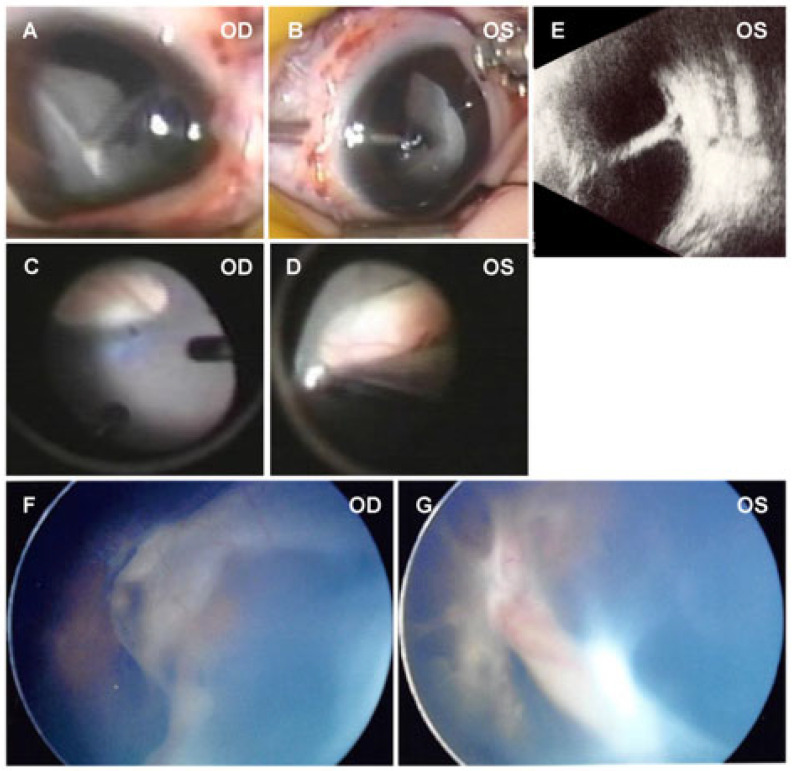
Preoperative and intraoperative fundus photographs of patients with mutations in the *DOCK6* gene. Intraoperative images and ultrasonographic images of Case #25 (A–E) showing retrolental fibrovascular proliferation in the periphery and dragged disk due to falciform retinal folds. Widefield fundus photographs of Case #26 (F,G) showing falciform retinal folds. OD, the right eye; OS, the left eye.
